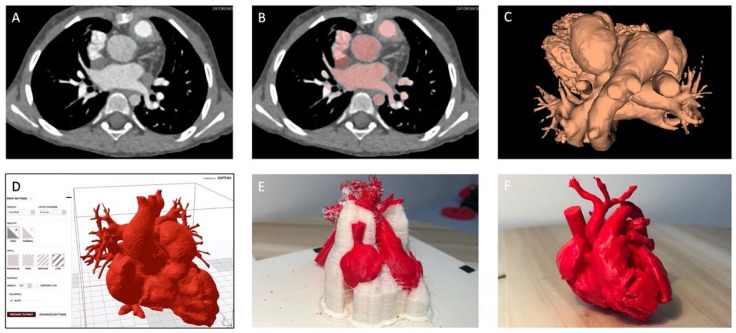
The steps in the process of 3D printing. (A). Source CT; (B). CT after segmentation (red); (C). Digital model (STL); (D). Print setup; (E). Raw print covered in support material; (F). Final 3D printed model. Detailed descriptions in text. (If not stated otherwise, images courtesy of Jarosław Meyer-Szary, Department of Pediatric Cardiology and Congenital Heart Defects, Faculty of Medicine, Medical University of Gdańsk, Gdańsk, Poland).
